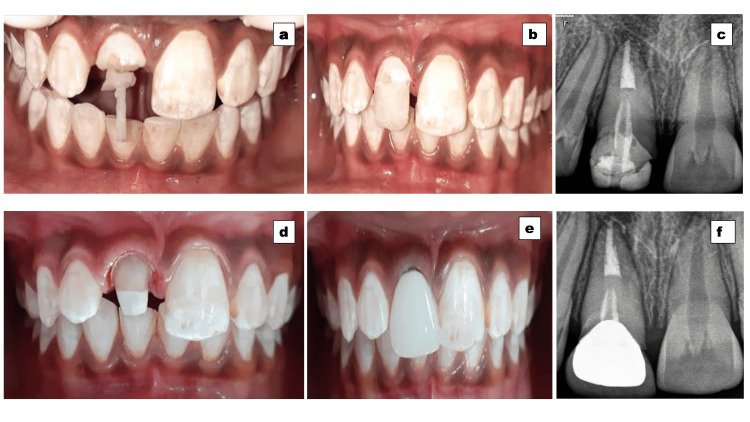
Cementation of the anatomic post. (a) Trial placement of the anatomical post within the canal. (b) Core build-up done. (c) Radiographic confirmation of post placement within the canal. (d) Crown preparation. (e) Final prosthetic restoration of the tooth. (f) Postoperative radiograph.
